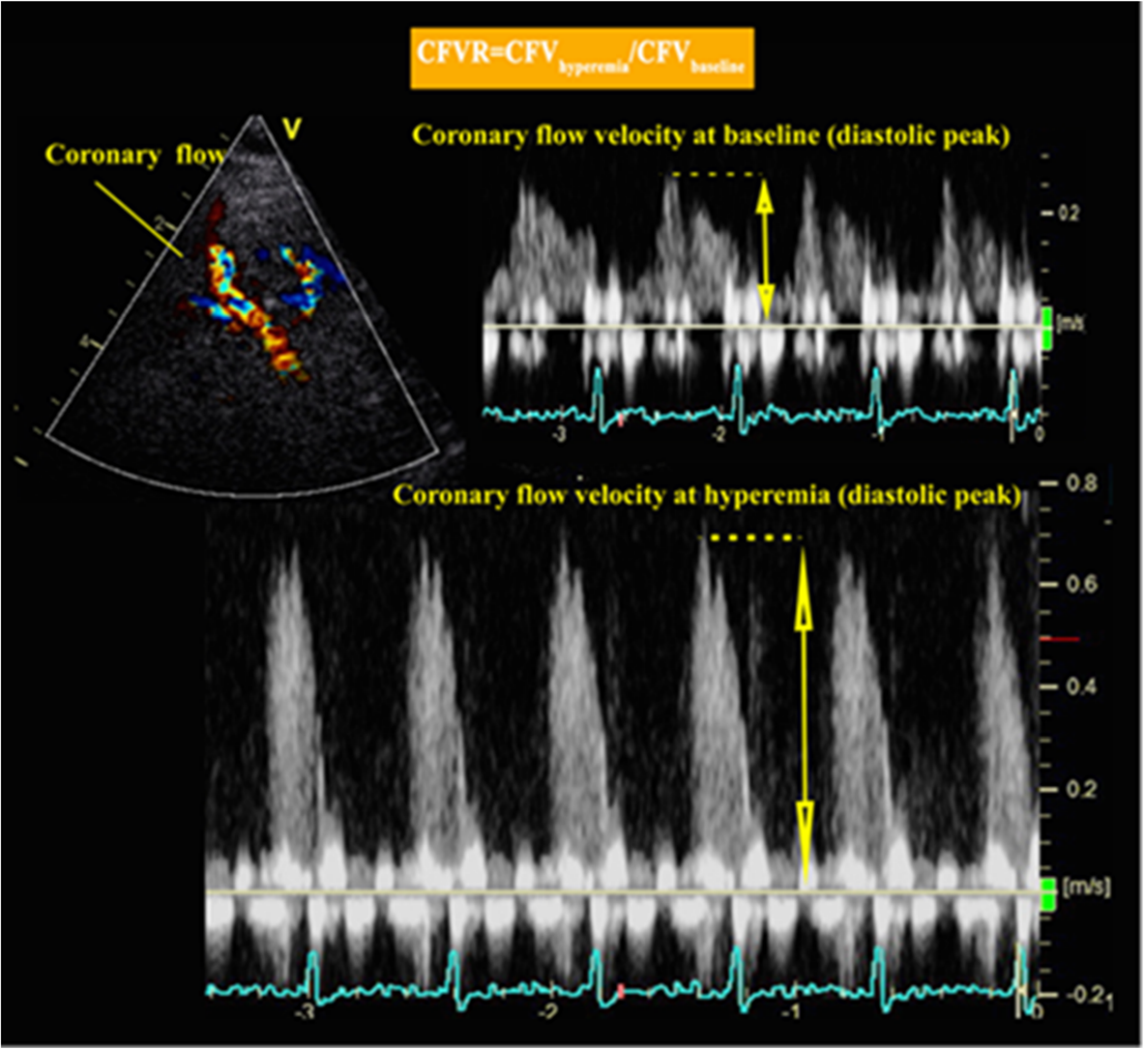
Measurement of coronary flow velocity by transthoracic Doppler echocardiography
